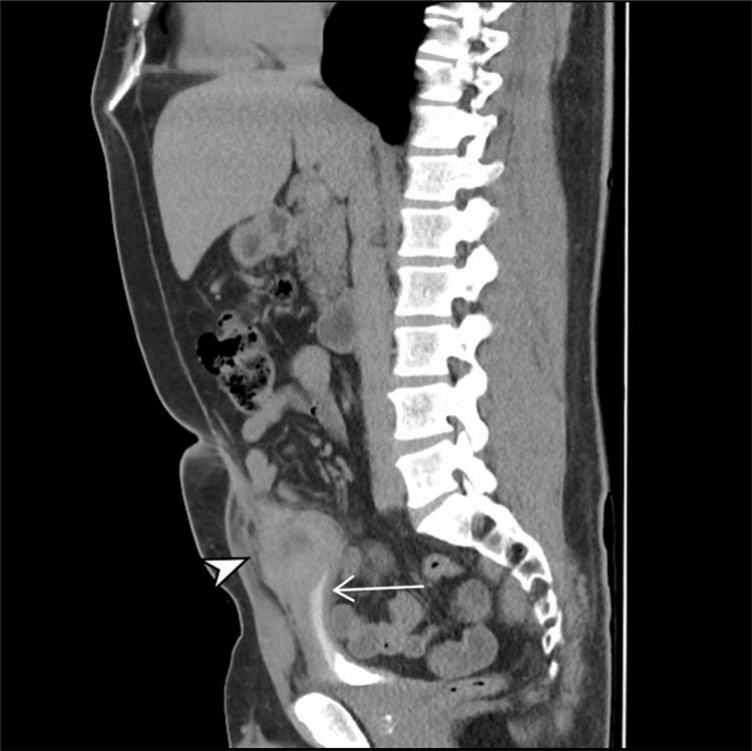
Sagittal CT cystogram. Arrowhead indicates involvement of anterior abdominal wall. Arrow indicates association of lesion with the dome of the bladder.
